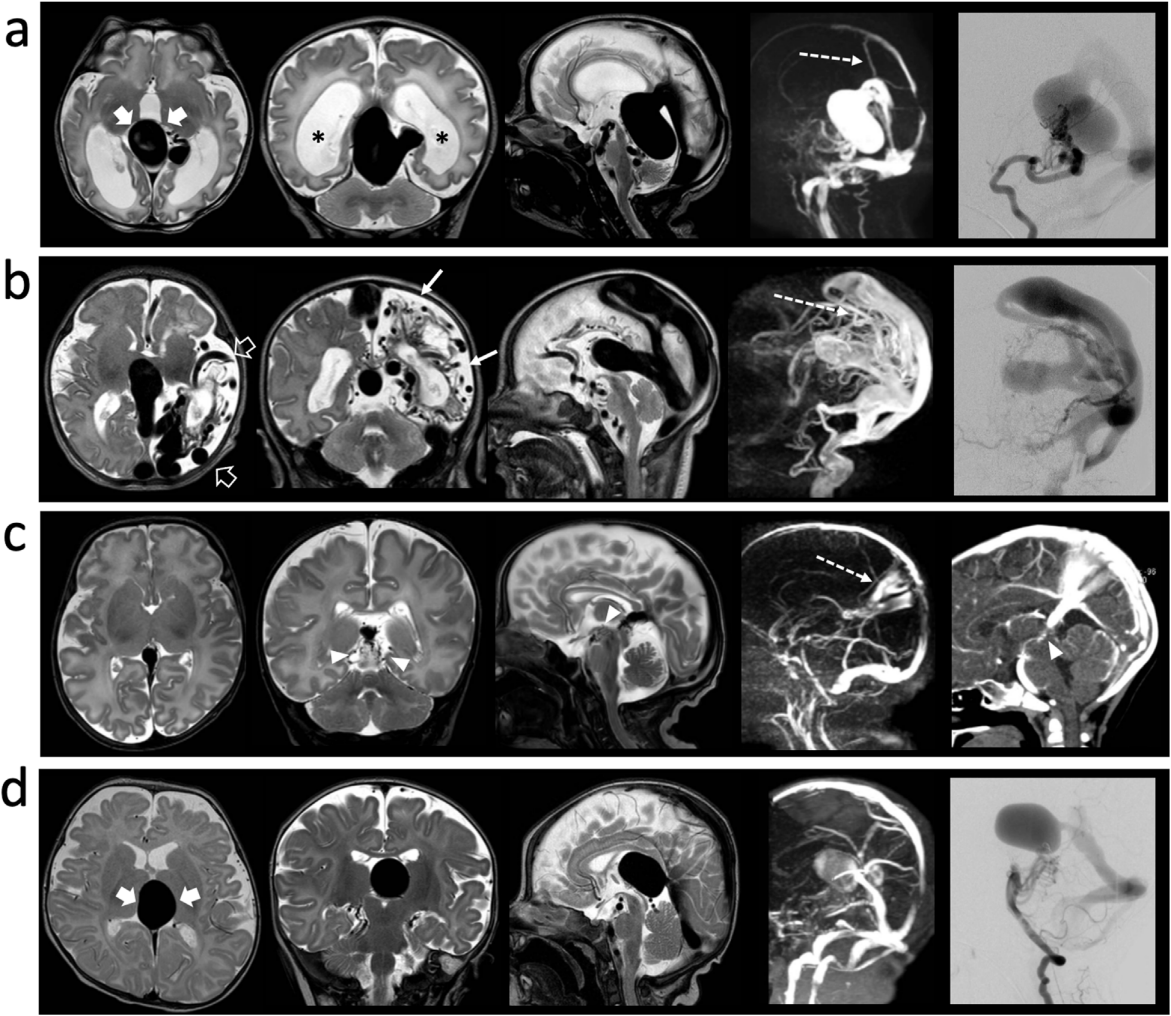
Neuroimaging features of (A) patient 1 and (B) patient 2 at birth, (C) patient 3 at 1.5 months of term-equivalent age, and (D) patient 4 at 3 months of age. Brain magnetic resonance imaging (MRI) examinations with axial T2-weighted (first column from left), coronal T2-weighted (second column from left), sagittal T2-weighted (third column from left), and venous MR angiography (fourth column from left); lateral views of (A, B, D) catheter angiograms (right column) and (C) CT angiography. Patients 1 and 4 show true vein of Galen malformations (VGAMs; thick arrows), while patient 2 shows a vein of Galen dilatation (VGD) secondary to a very large arteriovenous fistula in the right fronto-parietal region (empty arrows) and patient 3 shows a small arteriovenous malformation in the mesencephalon (arrowheads). Falcine sinuses are noted in patients 1, 2, and 3 (dashed arrows). We also note a marked ventricular dilatation with reduced white matter volume in patient 1 (asterisks) and a chronic ischemic infarct of the left hemisphere in patient 2 (thin arrows) that were present at birth.
